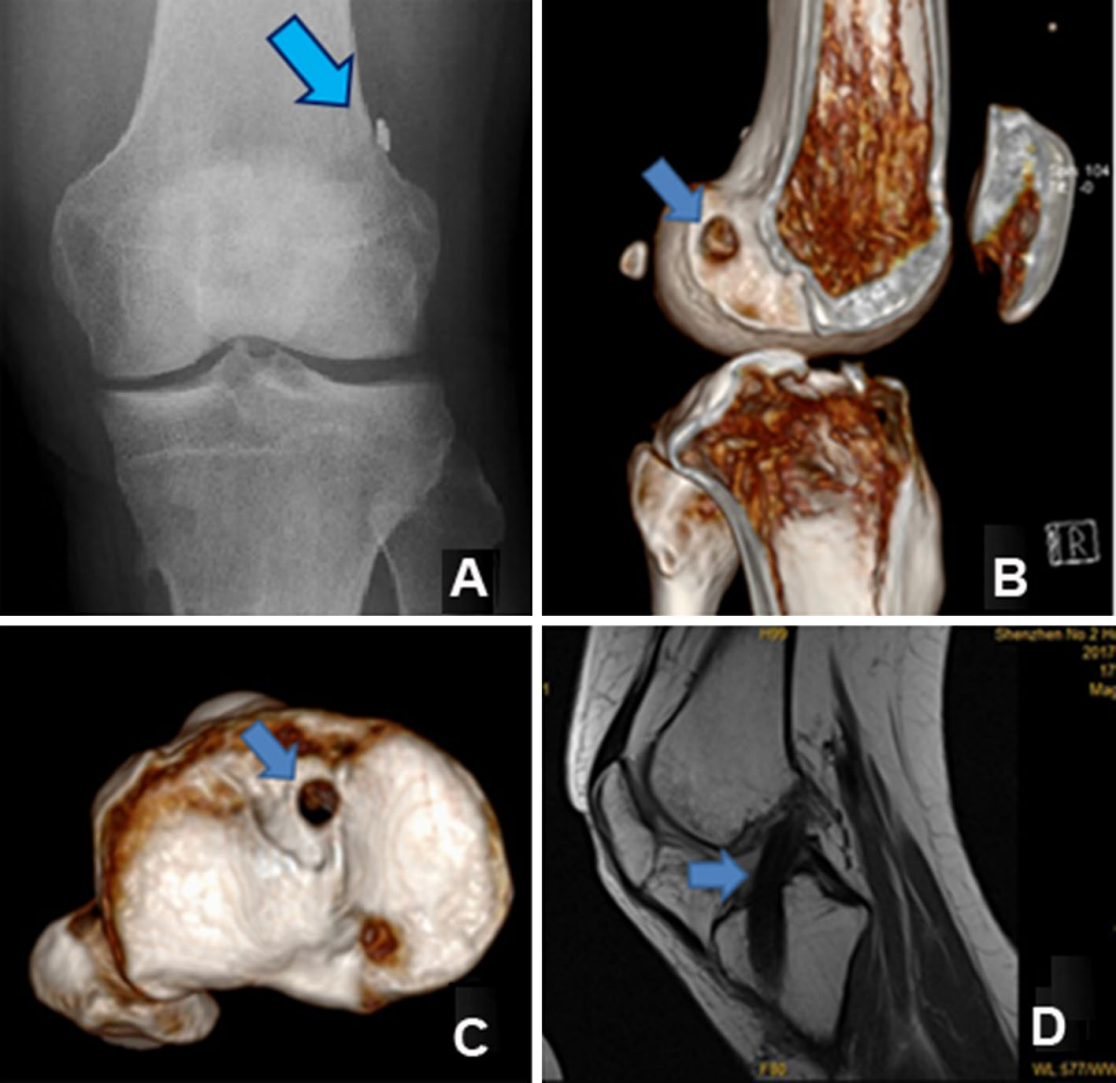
Postoperative radiological examination of single-bundle ACL individualized anatomical reconstruction after over 2 years. (A) X-ray: arrow shows the fixation of Endobutton. (B) 3D CT: arrow shows the femoral socket. (C) 3D CT: arrow shows the tibial socket. (D) MRI: arrow shows the graft.
